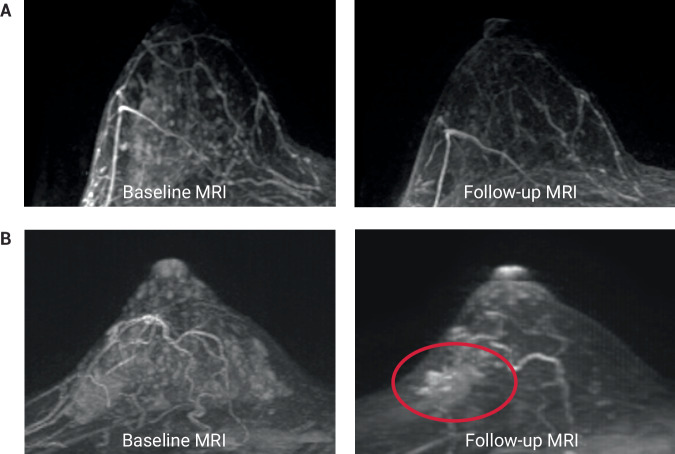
Changes in ipsilateral breast MRI features. Axial subtracted post-contrasts maximum intensity projection (MIP) images of the ipsilateral breast before and after endocrine therapy. One example shows marked BPE at baseline that is reduced after endocrine therapy with no distinct lesion prior to or following ET (A), and the other shows marked BPE with no distinct lesion above BPE at baseline and a reduction in BPE after endocrine therapy and a distinct lesion on follow-up (oval) (B).
